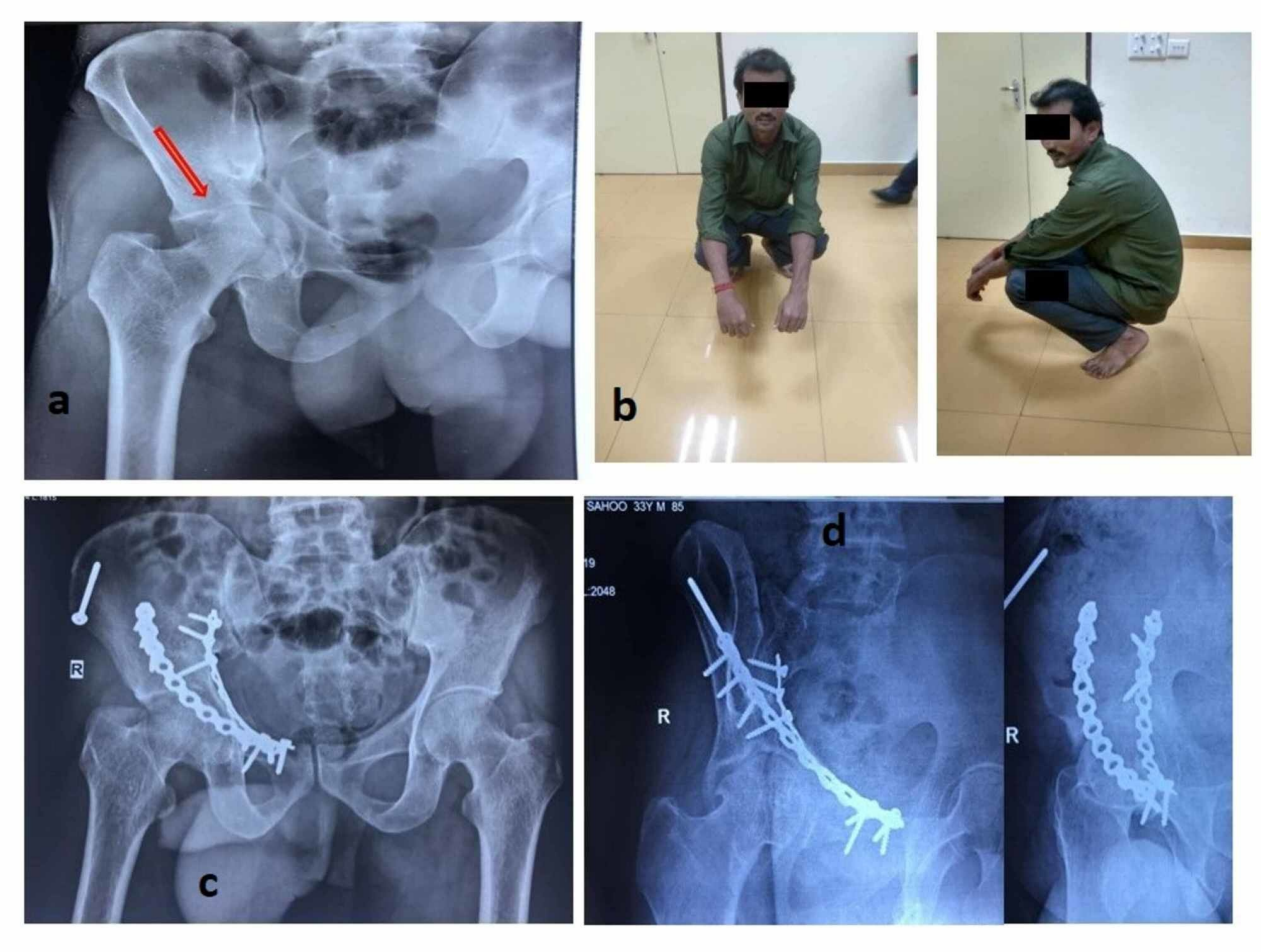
A case of transverse fracture showing head impaction (red arrow) with final postoperative X-rays and functional outcome a: preoperative radiograph; b: clinical functional outcome; c: follow-up radiograph anteroposterior view; d: follow-up radiograph iliac and obturator view
